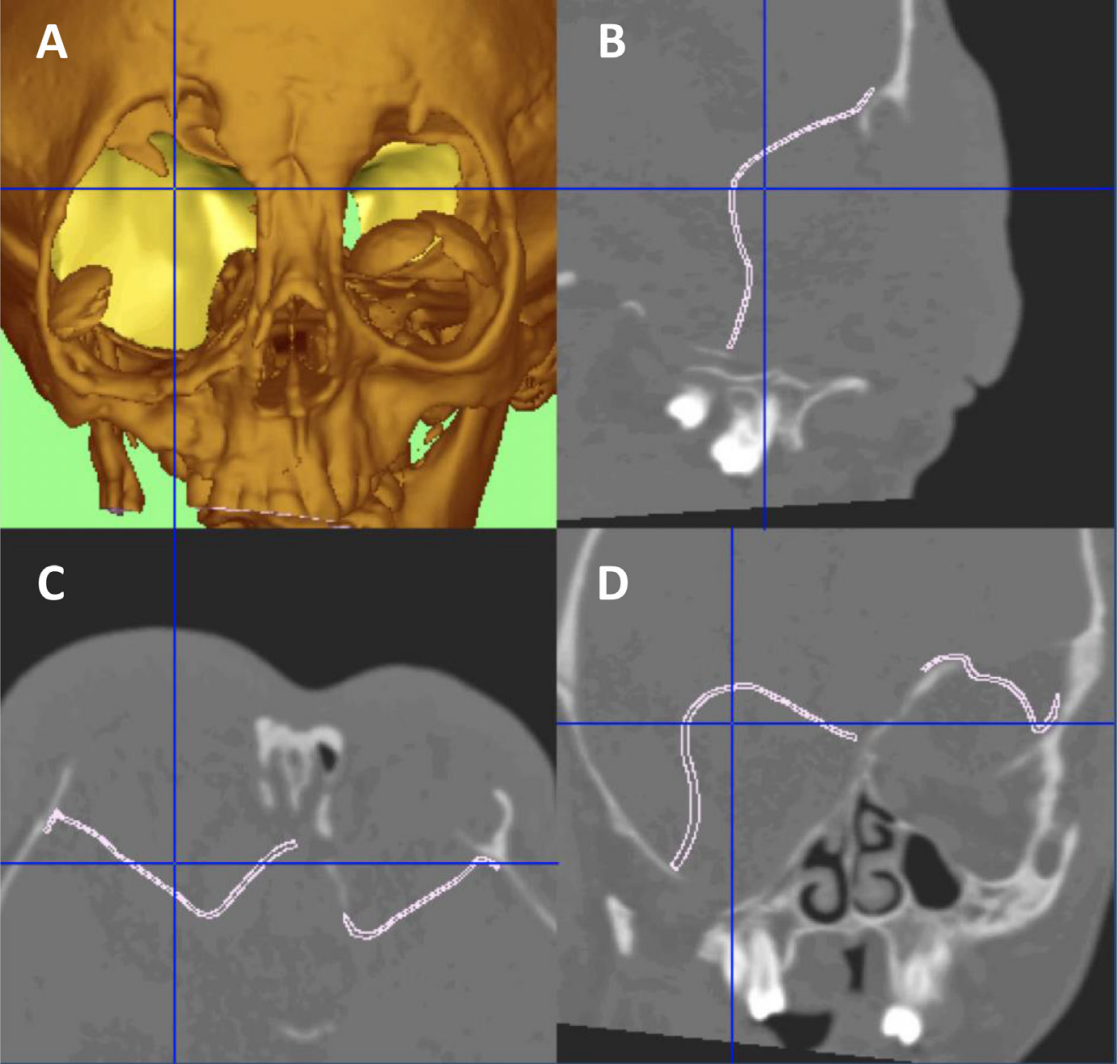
(A) 3D CT reconstruction of the sphenoid wing dysplasia defect. (B, C, D) CT planning of the custom-made titanium implants.
